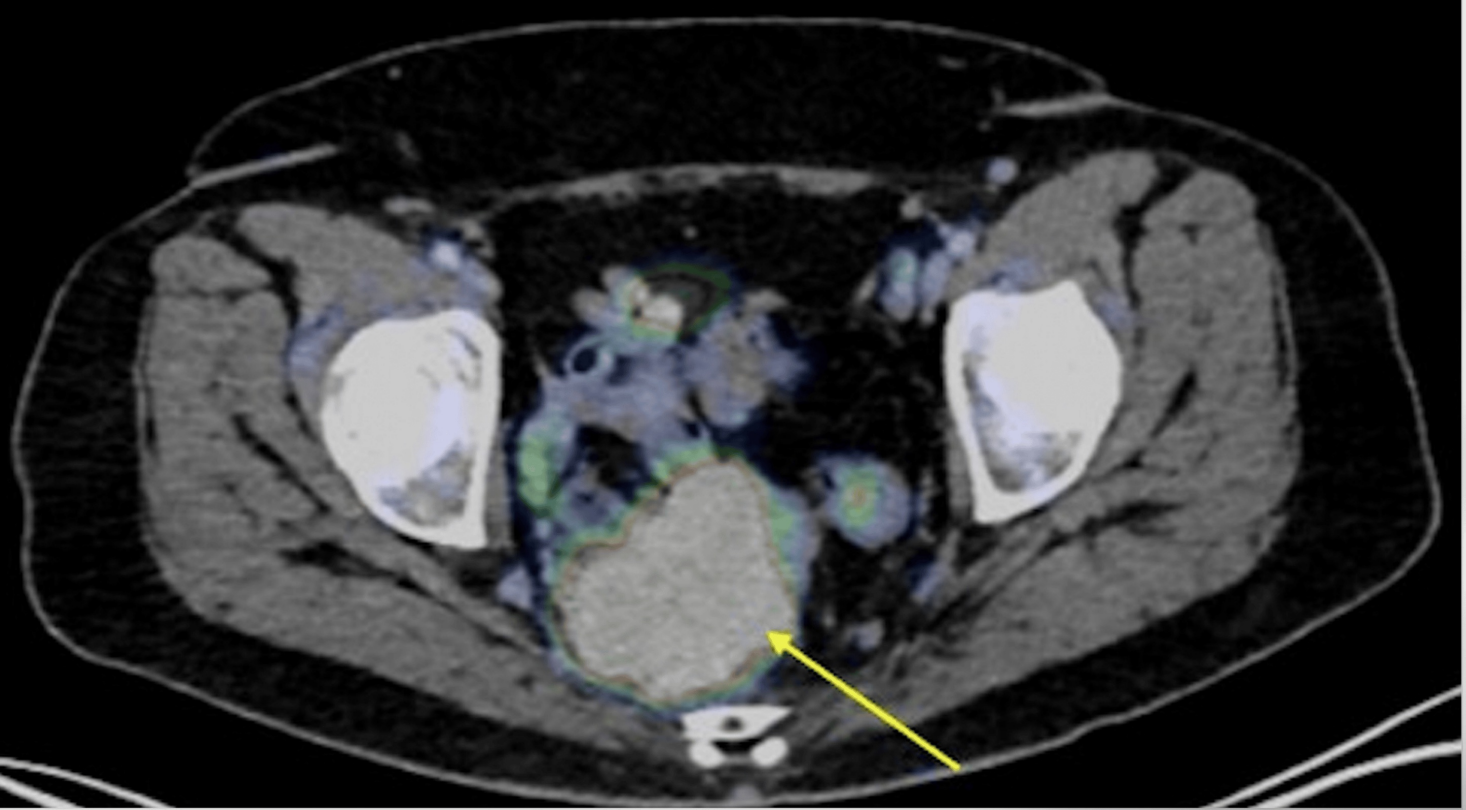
Axial fused PET/CT image demonstrating a large presacral mass (yellow arrow) with concern for serosal bowel invasion. PET/CT: positron emission tomography/computed tomography
